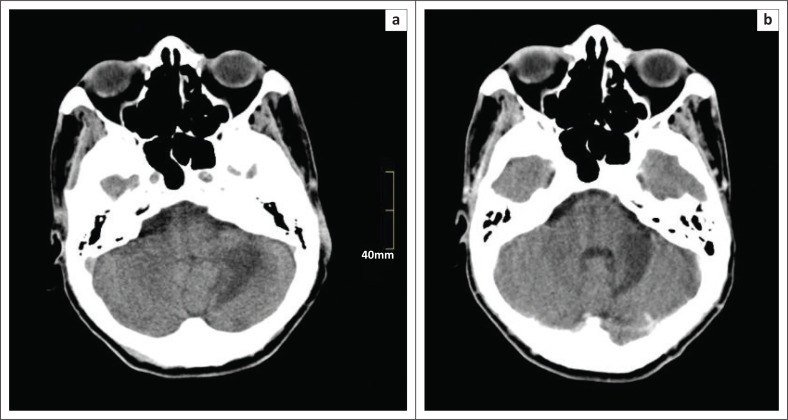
Axial (a) pre- and (b) post-contrast CT scan of the patient discussed in Case 5, demonstrating low density in the left cerebellar hemisphere with no contrast enhancement of the lesion.
